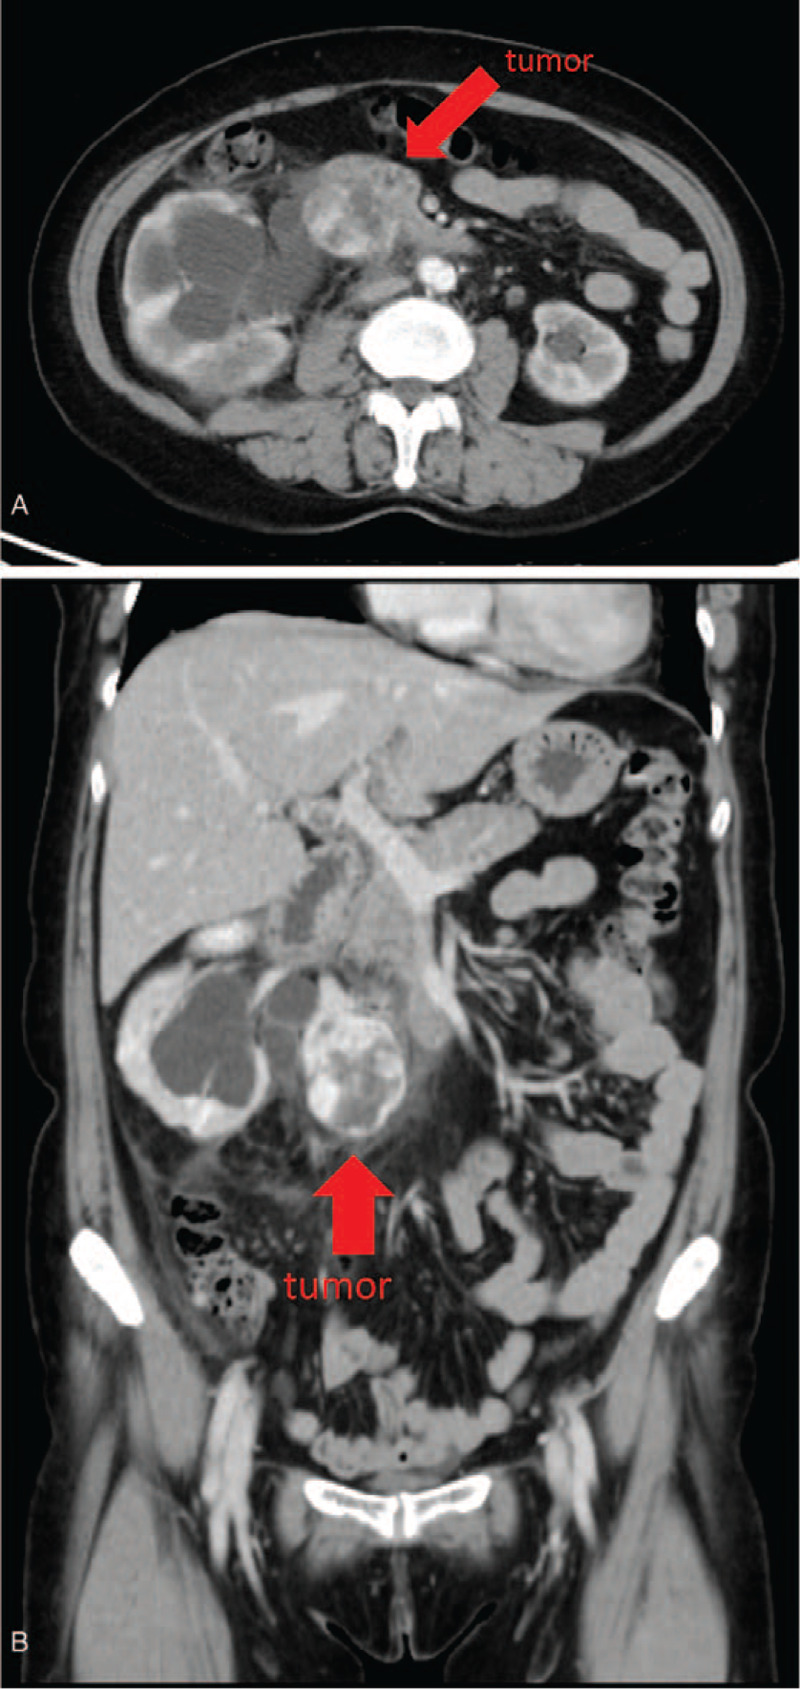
CT scan showed heterogeneous enhancement over the right retroperitoneum. (A) Transverse view, (B) coronal view. CT = computed tomography.
